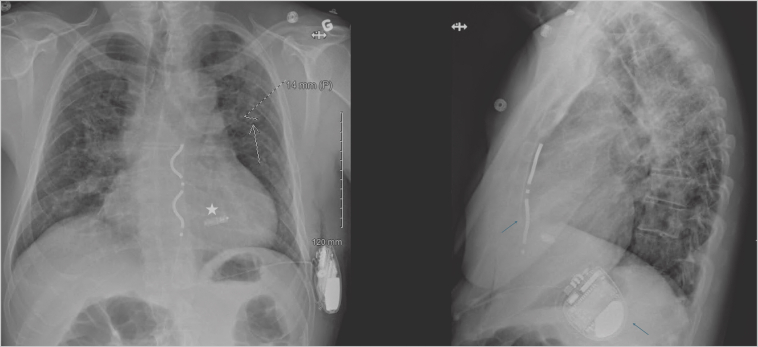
Face and lateral chest radiograph showing the Micra pacemaker (*whitestar*) and the substernal extravascular implantable cardioverter-defibrillator system (*blue arrows*).
